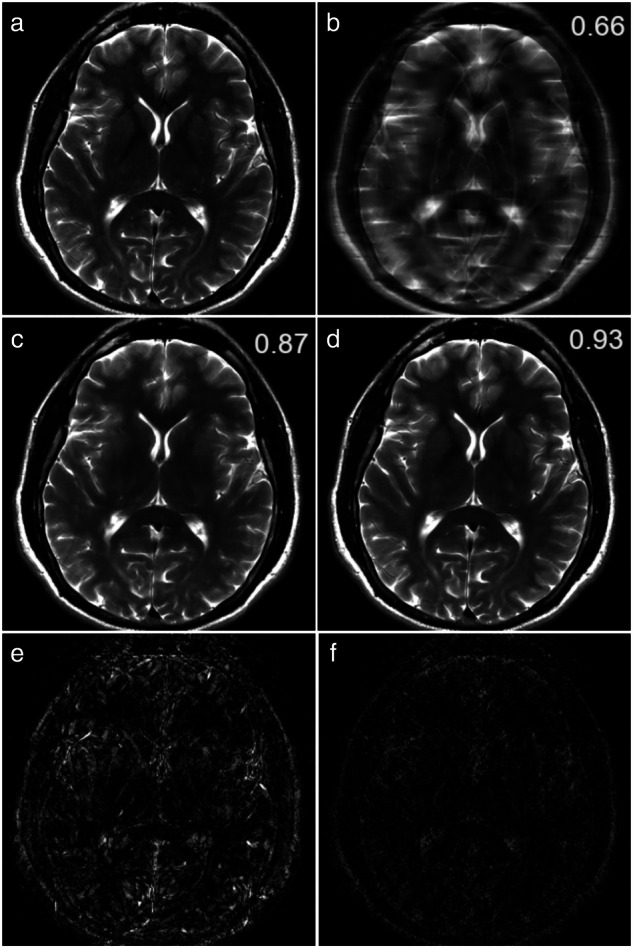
Results of CNN-based algorithms for image enhancement that work on either raw data or on already-reconstructed images. a Brain T2-weighted reference image, axial scan at the basal ganglia level, fully sampled. b Four-times accelerated image, zero-filled. c Output from the postprocessing algorithm. d Output from the raw-data algorithm. e Absolute errors for panel (c). f Absolute errors for panel (d). The structural similarity index (range 0–1) is displayed in white at the top right of every image. Reproduced with permission [15]
